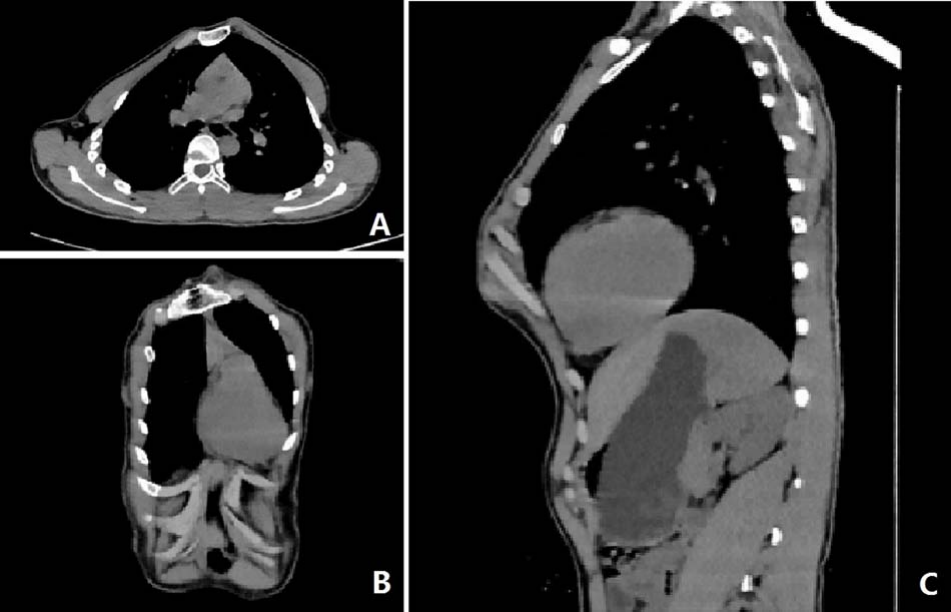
CT examination images before operation.
